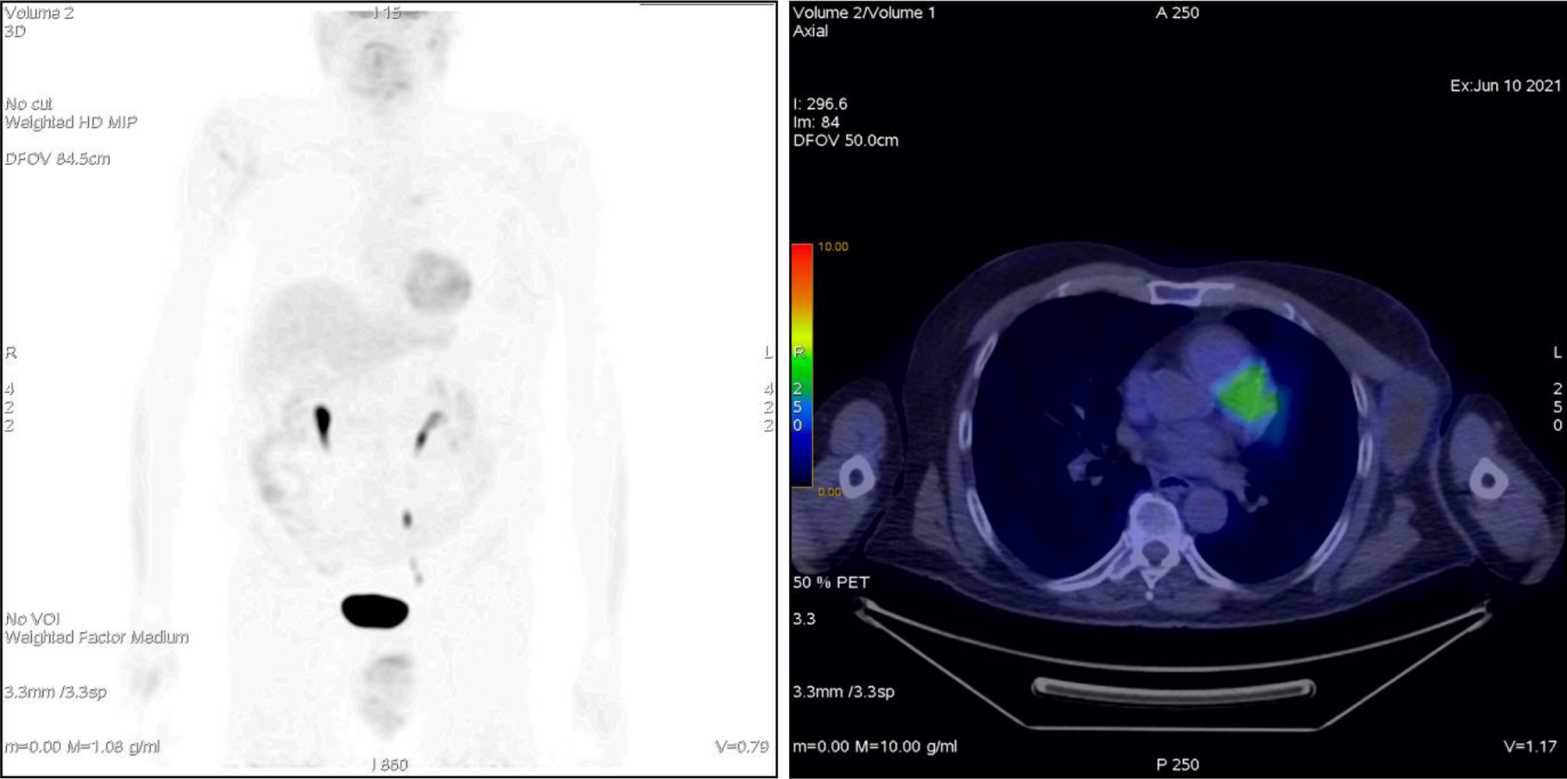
Patient ^18^F-FDG PET 1 month after treatment.
